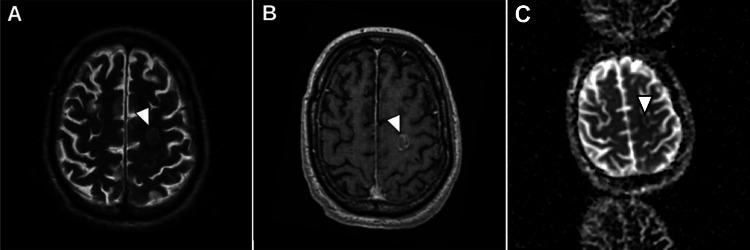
Cerebral magnetic resonance images Images A and B: Arrowheads pointing to a cortical lesion on the superior left frontal gyrus with a hypersignal in the T2 sequence with peripheral enhancement.
